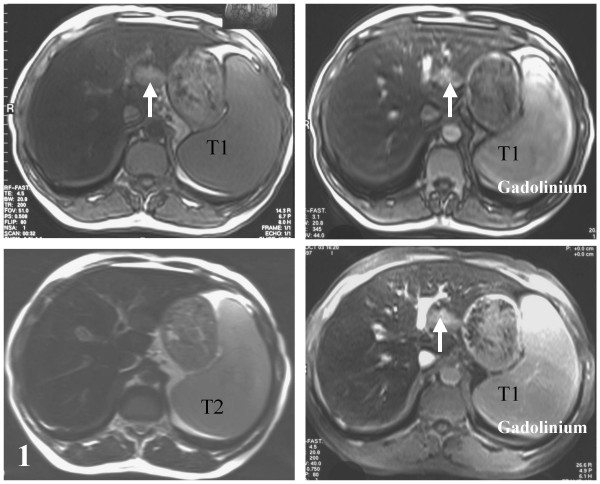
Hyperintensity of the nodule on T1- and T2-weighted images (left), which remains so after gadolinium injection (right), on T1-weigthed image in the portal phase.
